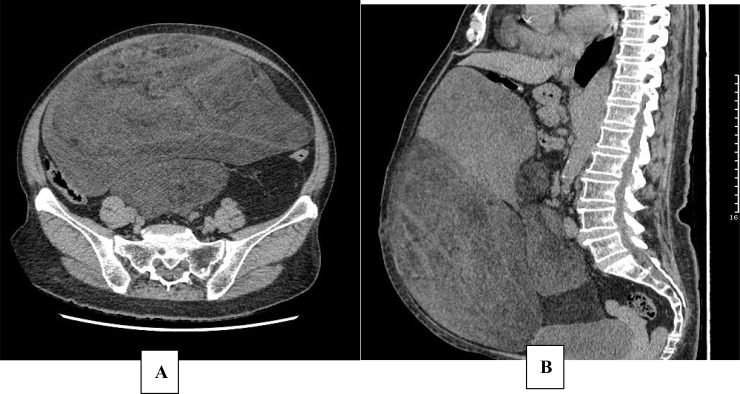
Abdominal CT. Abdominal CT obtained in August 2020 (after anlotinib therapy). Stable disease (SD) confirmed by imaging data. Axial (A); Sagittal (B).
